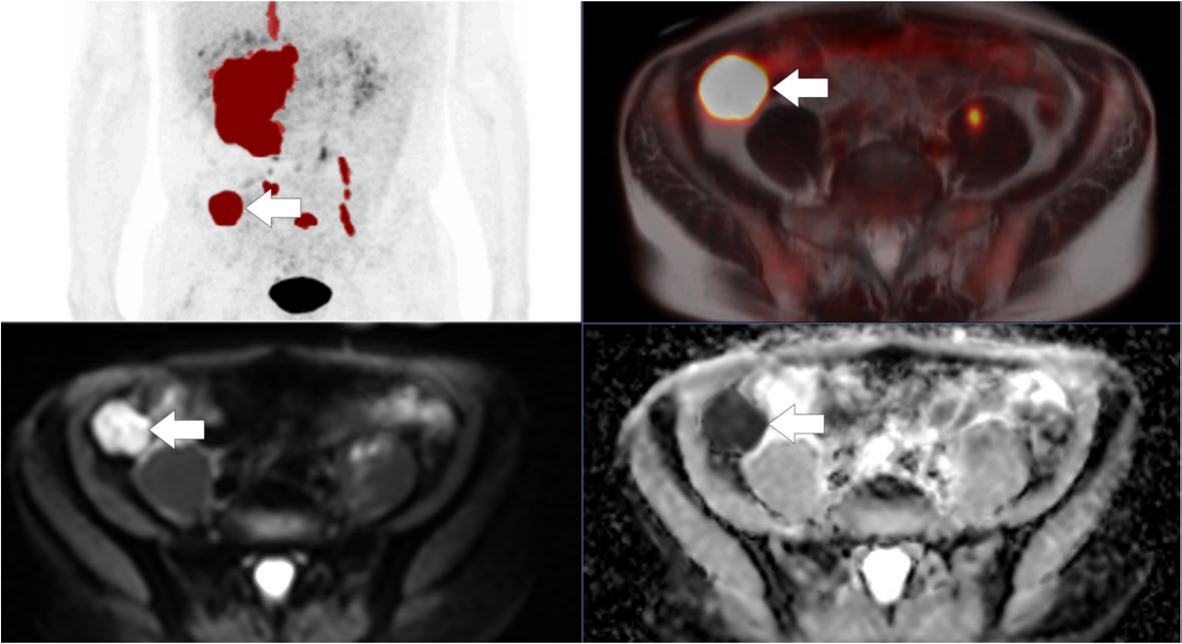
Baseline PET/MRI of a 64-year-old male with Diffuse Large B-cell Lymphoma, stage IVBX and IPI 4 in complete remission 2 years after baseline staging. Metabolic tumor volume was 563 cm^3^. The region of interest was a 30x33mm FDG avid target lesion in the right pelvic/iliac region. Minimum apparent diffusion coefficient (ADC_min_) was 165 mm^2^/s and mean apparent diffusion coefficient (ADC_mean_) was 545 mm^2^/s. Upper left: PET MIP MTV. Upper right: Fused PET with T2-HASTE. Lower left: Diffusion weighted imaging (b800). Lower right: ADC-map
